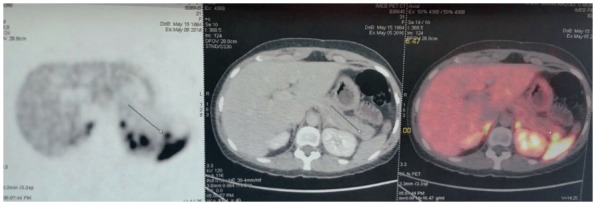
Octreotide scintigraphy showing a pancreatic tail neuroendocrine
